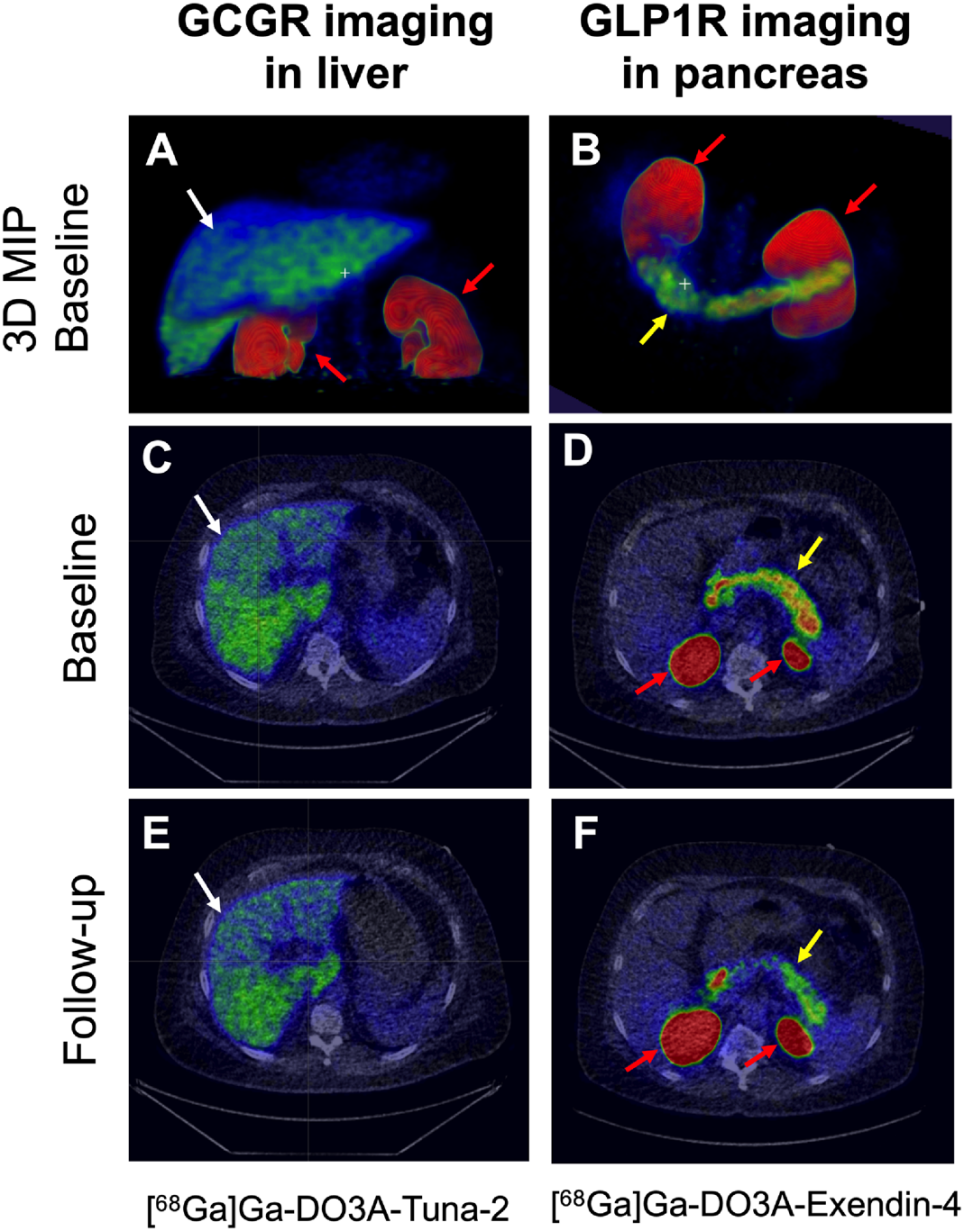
Representative images (A, B: 3D Maximal Intensity Projections (MIP) and C–F: trans-axial projection) of PET uptake for the tracer for the GCGR (left panels, A, C, E) and GLP1R (right panels, B, D, F). [^68^Ga]Ga-DO3A-Tuna-2 displayed strong binding in the liver which is rich in GCGR combined with low or negligible uptake in surrounding GCGR negative tissues (A, C). [^68^Ga]Ga-DO3A-Exendin-4 on the other hand showed strong binding to GLP1R in pancreas at baseline (B, D). Both PET tracers were excreted through renal route and trapped in the kidney cortex. The reduction in binding at the follow up examinations following treatment are proportional to the SAR425899 drug occupancy at the respective receptor (E, F). The baseline and follow-up images for the respective tracers are normalized to SUV = 5 and are directly comparable. White arrows indicate liver, yellow arrows indicate pancreas and red arrows indicate kidneys.
